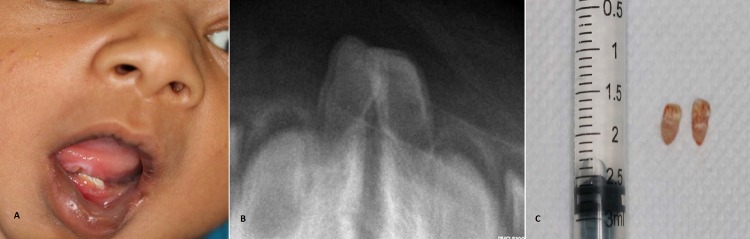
Figure 1: A) Natal teeth seen in the lower arch and ulcer seen on the ventral side of tongue. B) Intraoral radiograph showing two natal teeth in mandibular anterior region. C) Extracted Natal teeth.
